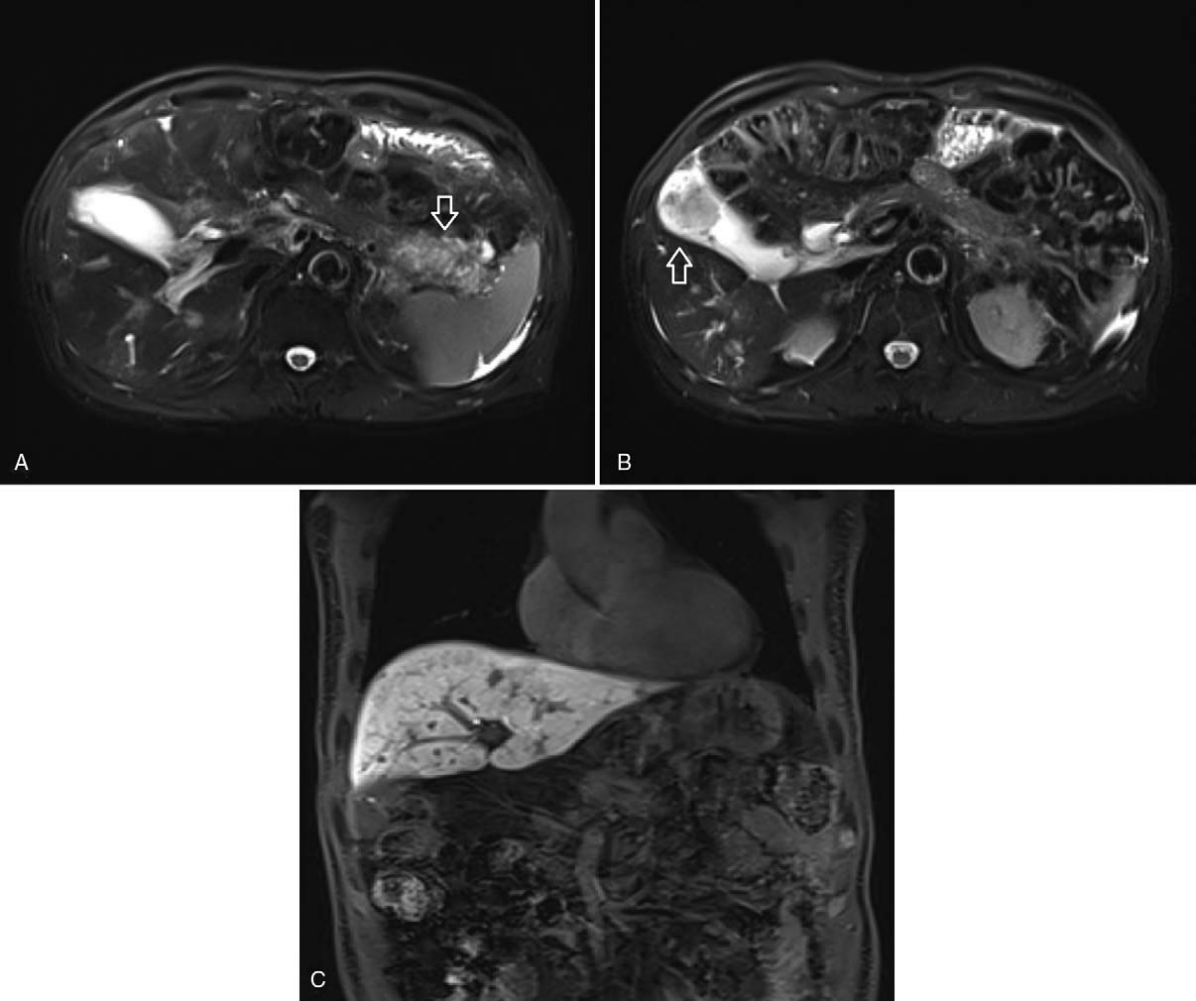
Pancreas MRI. (A) A 62 × 40 mm delayed enhancing mass in the tail of the pancreas with splenic vessel invasion (arrows). (B) A 37 × 34 mm heterogeneously enhancing mass in the gallbladder (arrows). (C) Multiple hepatic metastases. MRI = magnetic resonance imaging.
